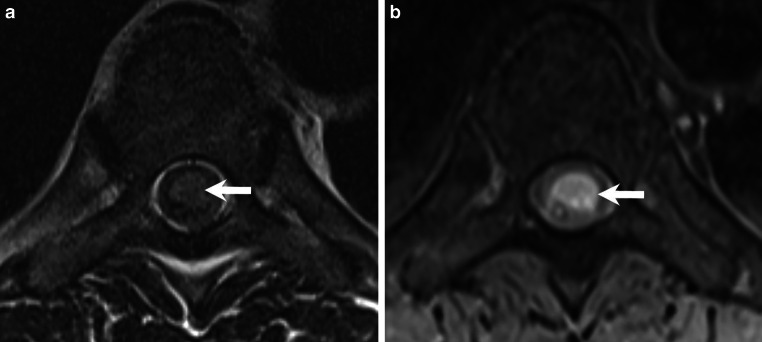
Cross-sectional images at the Th8 level show a slightly hyperintense signal on axial T2-weighted images (a, arrow) and a marked hyperintense signal on T1-weighted images without contrast (b, arrow) in the space-occupying portion of the lesion
